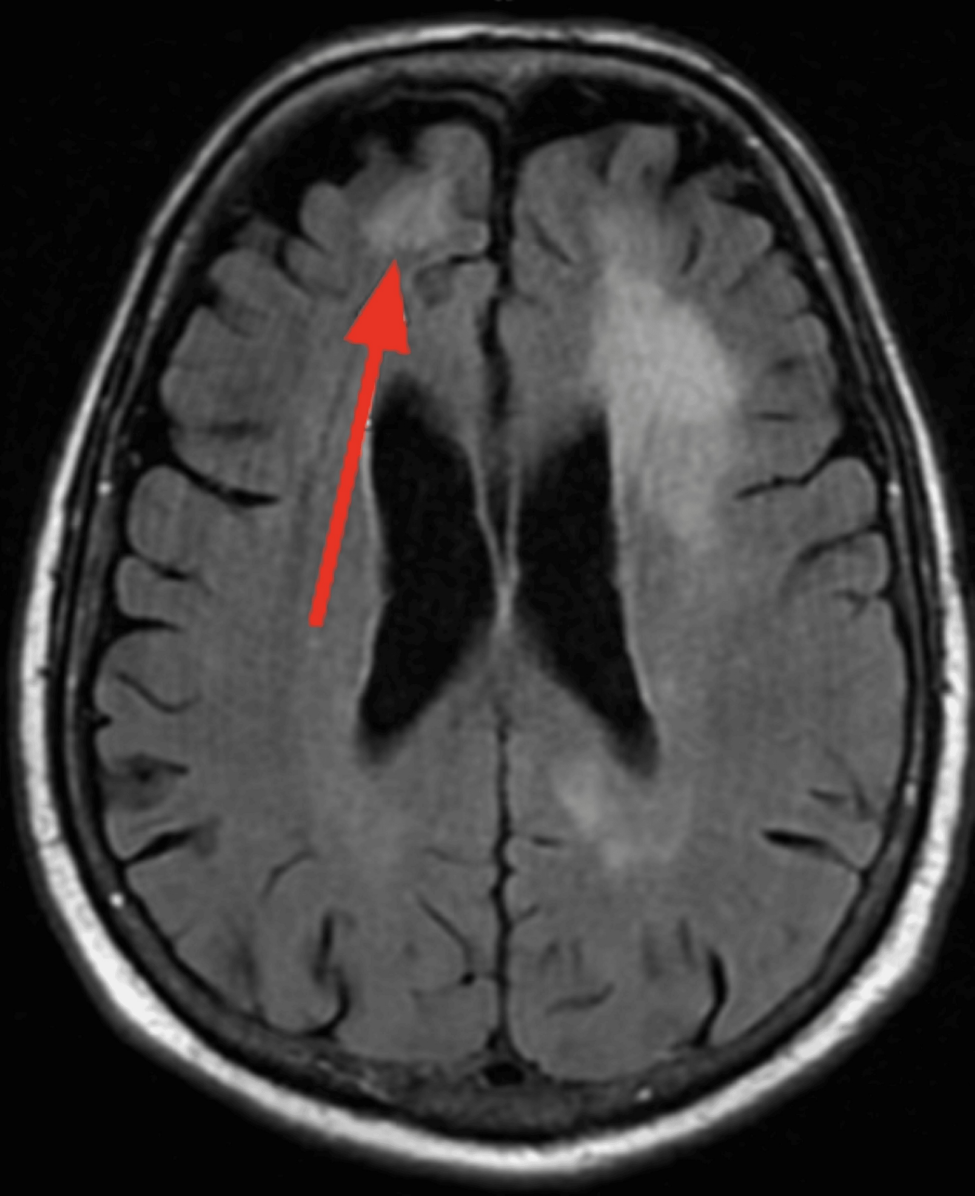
MRI of the brain showing an area of hypodensity in the left frontal lobe, marked by the arrow.
